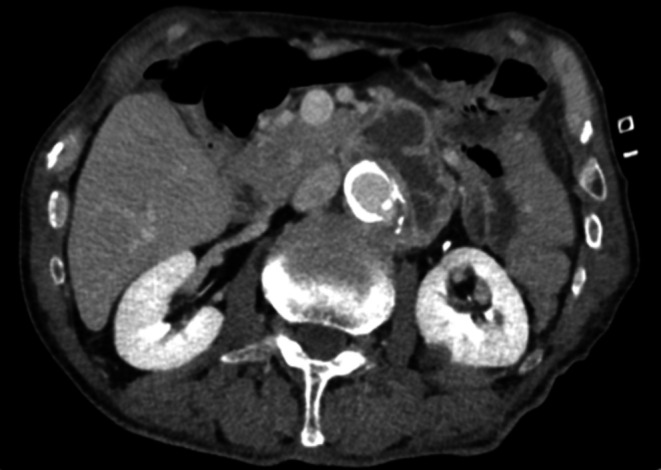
Contrast‐enhanced computed tomography at admission. Axial view showing a saccular infrarenal abdominal aortic aneurysm with marked periaortic inflammation and left iliopsoas abscess.
